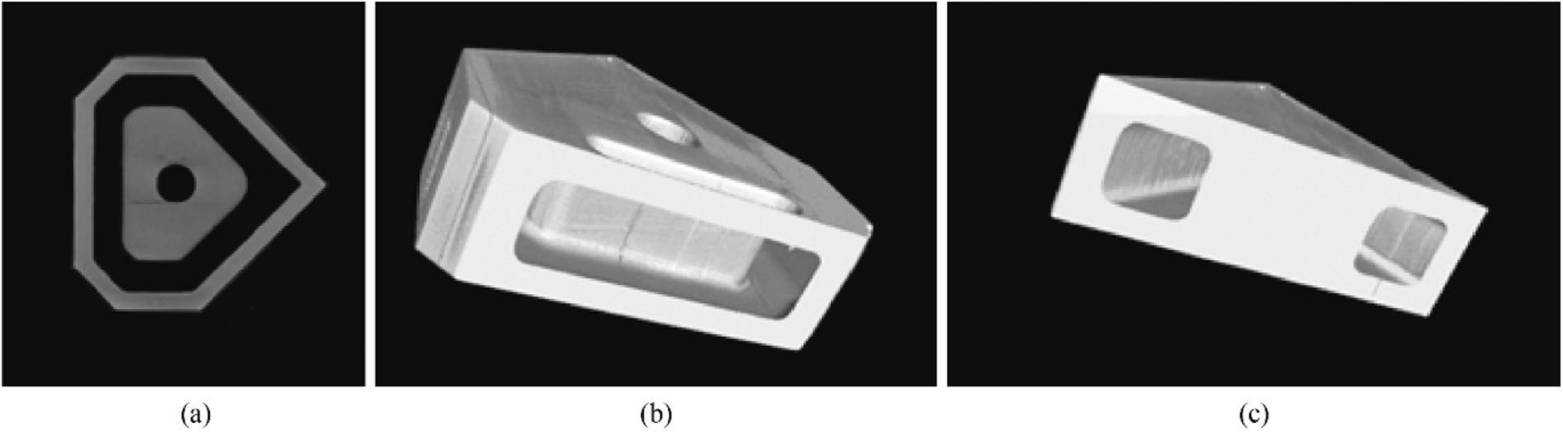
a Digitised CT image slice with CAD geometry overlapped for comparison (light green), b, c, time lapsed dynamic 3D CT image of insert
